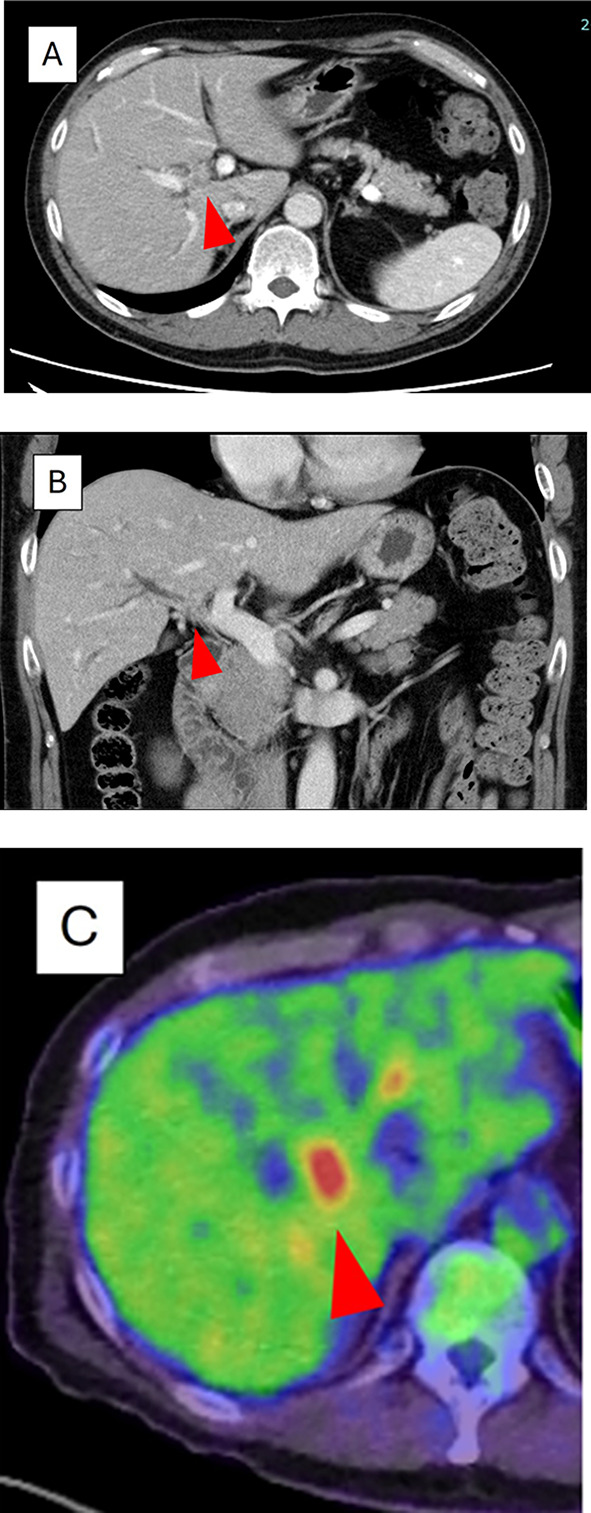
Preoperative imaging. A Contrast-enhanced abdominal CT (axial view) showing a contrast-enhanced mass at the hepatic hilum (arrowhead). B Coronal view of the same lesion as in (A) (arrowhead). (C) FDG-PET demonstrating increased uptake in that region (arrowhead)
